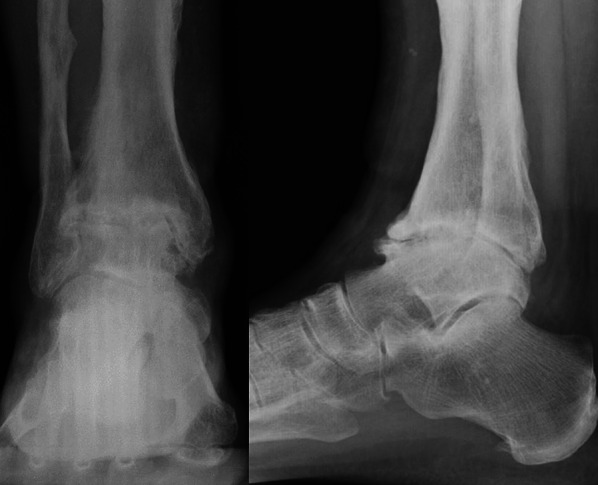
Anteroposterior and lateral weight-bearing X-rays of patient showing severe ankle arthritis
